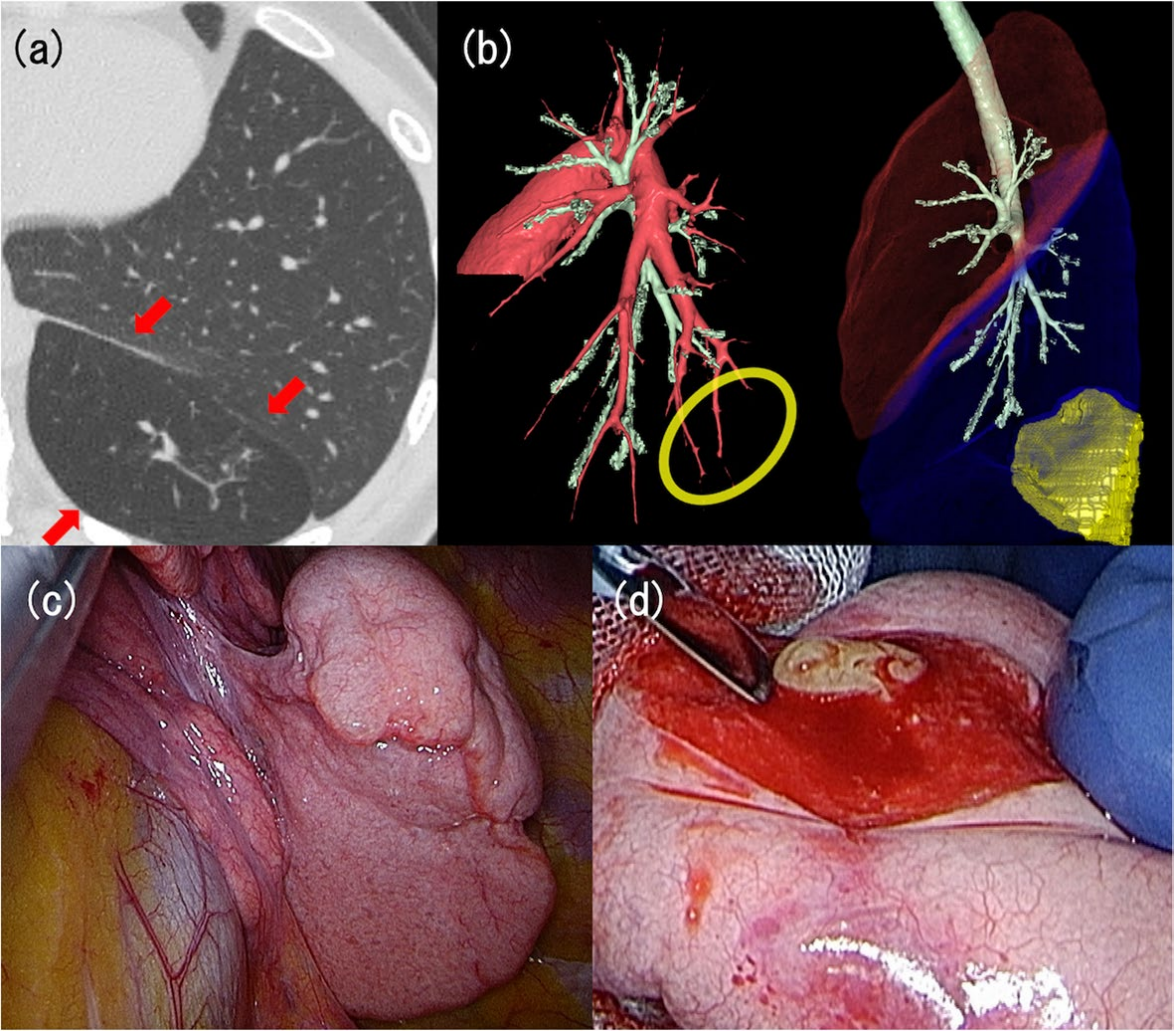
Chest computed tomography (CT) images and intraoperative findings of case 1. a Chest CT indicated the abnormal low-density area with an aberrant fissure in S9 and S10 in the left lung, as indicated by red arrows. b Two images obtained from three-dimensional reconstruction of chest CT findings: In the left image, red and green structures represent pulmonary arteries and bronchi, respectively. The yellow ellipse represents the abnormal region. In the right image, the red zone is the left upper lobe, and the blue zone is the left lower lobe. The green structures represent the bronchi. The yellow area indicates the lesion. Two images revealed no bronchi to the lesion. c The lesion attached to the normal lung by a peduncle. d Yellow mucus in the resected lung
